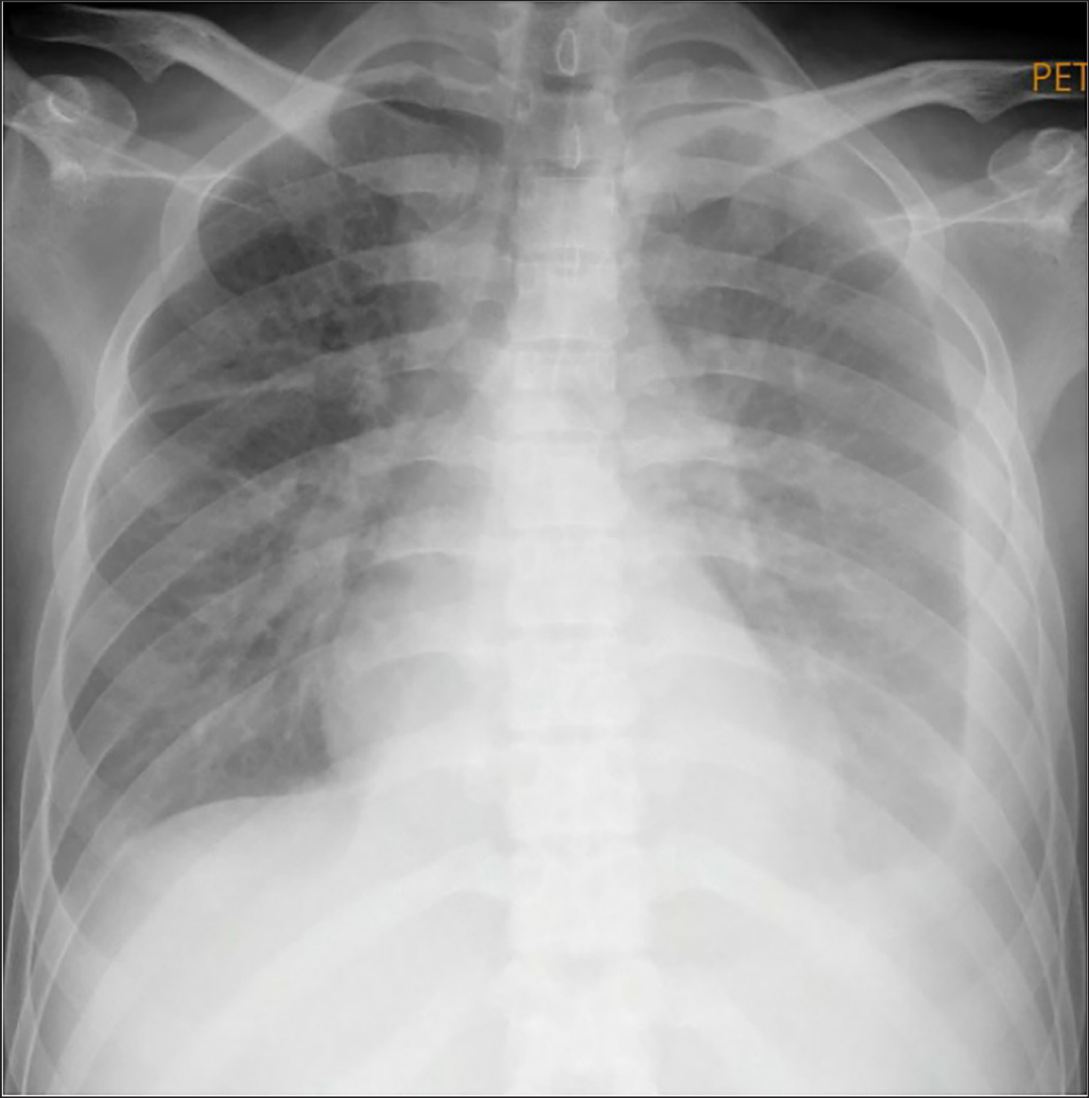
Chest radiographic findings demonstrated bilateral pleural effusion.
